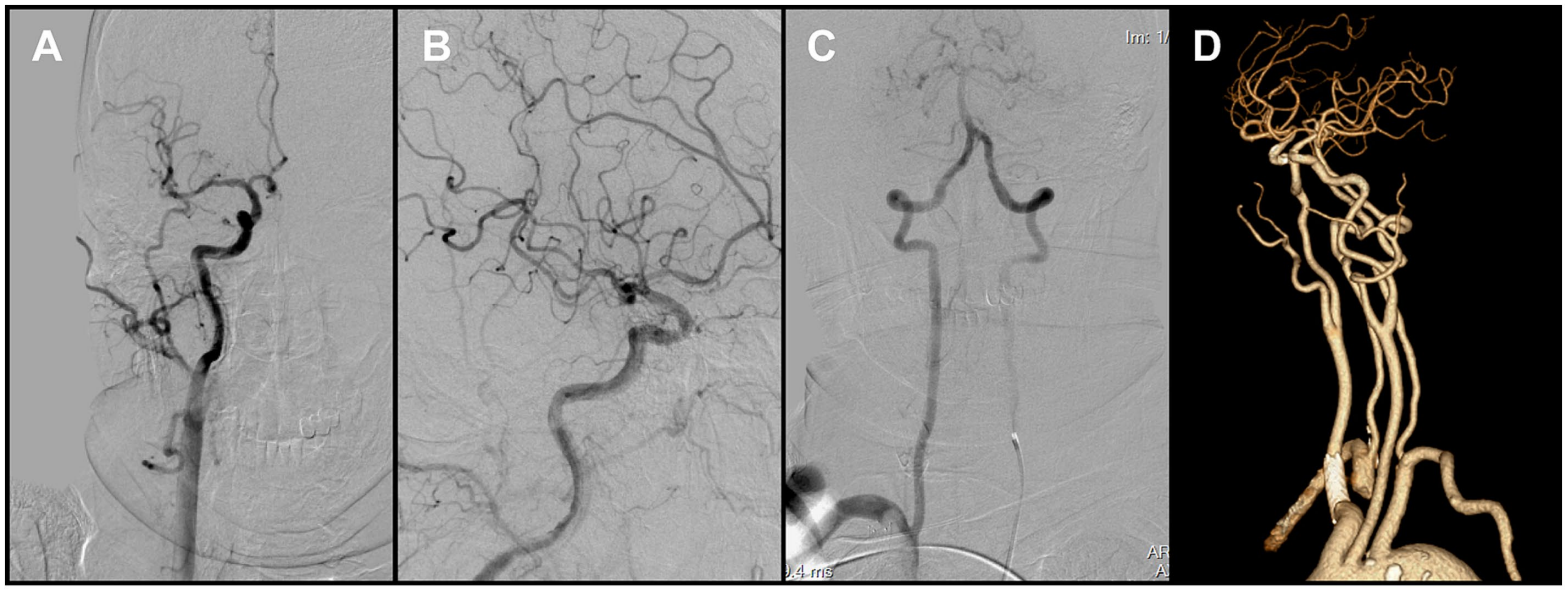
(A,B) Final angiography prior to procedural completion demonstrated reperfusion of the right carotid system (mTICI grade 2C). (C) Left vertebral angiography showed retrograde flow in the right vertebral artery. (D) Follow-up CTA at 1 month demonstrated persistent occlusion of the right subclavian artery.
